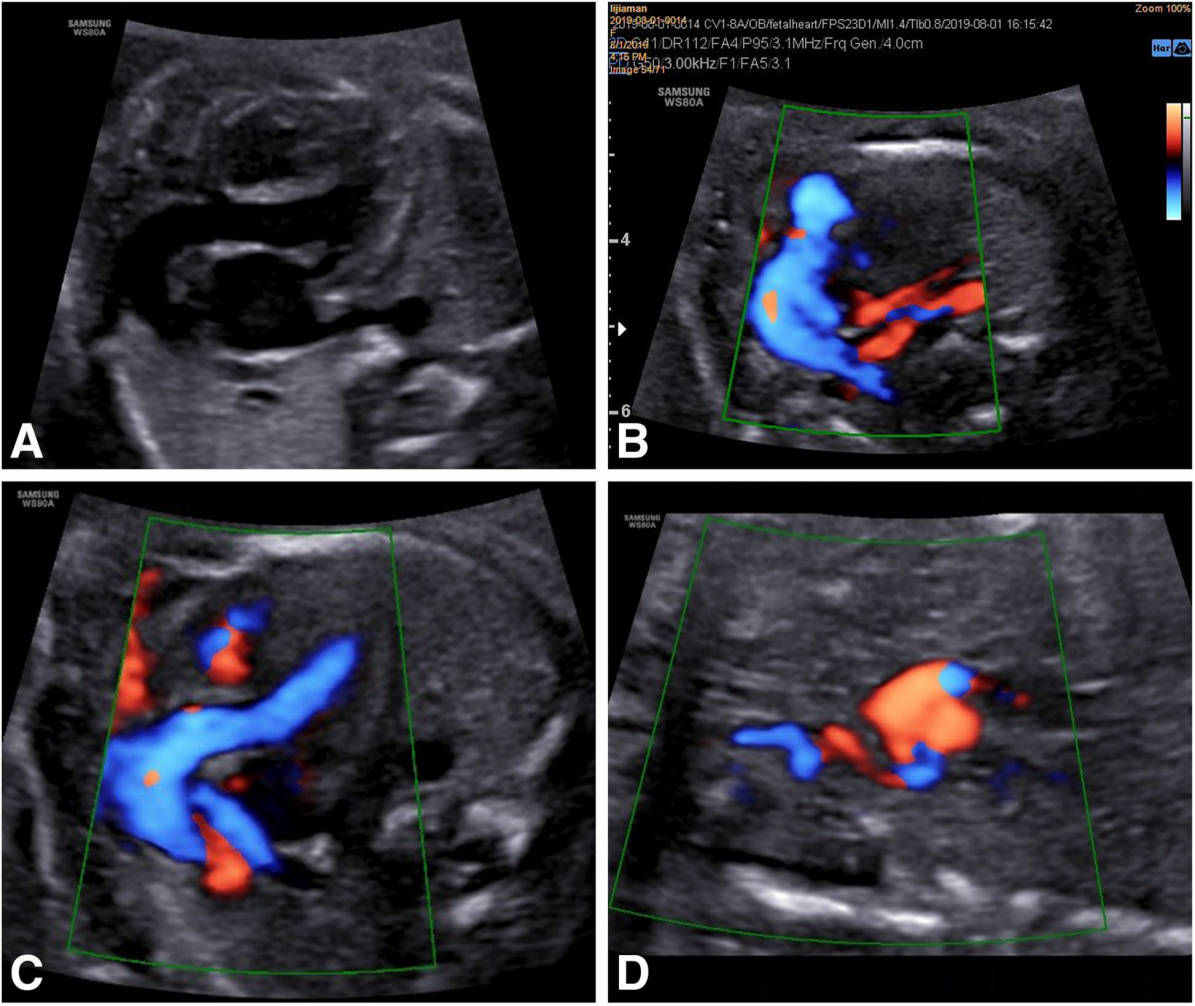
The prenatal ultrasonic image of the second fetus at 22 weeks. A, C Showing the tortuous and elongated aorta that similar to the images of the first fetus. B RPA, LPA and DA are significantly elongated and tortuous. D RCA is tortuous originated from IA. The measurement in detail as follows: AAO 0.46 cm, Aortic Arch 0.47 cm, MPA 0.59 cm, RPA 0.18 cm, LPA 0.19 cm, DA 0.19 cm, RCA 0.19 cm. AAO: ascending aorta; DA: ductus arteriosus; MPA: main pulmonary artery; RPA: right pulmonary artery; LPA: left pulmonary artery; RCA: right common carotid artery
